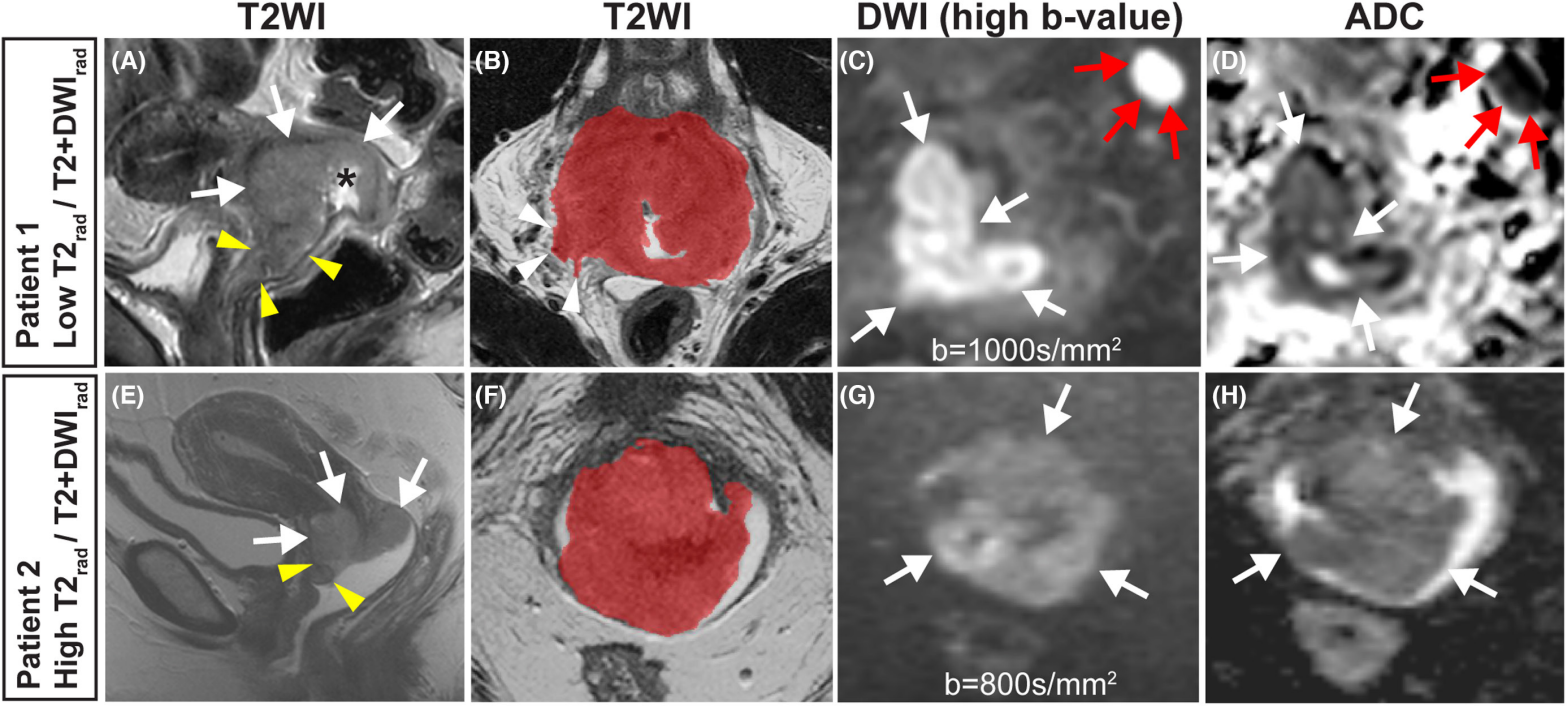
Cervical cancer depicted by sagittal T2‐weighted imaging (T2WI) (A, E), axial oblique T2WI (with manually segmented tumor mask) (B, F), axial/axial oblique diffusion‐weighted imaging (DWI) (*b* = 1000 s/mm^2^ and *b* = 800 s/mm^2^) (C, G) with corresponding apparent diffusion coefficient (ADC) maps (D, H) in two different patients diagnosed with 2018 FIGO stage IIIC1. (A–D) Patient 1: A 48‐year‐old woman having low radiomic score for the signatures T2_rad_ and T2 + DWI_rad_ and a large cervical tumor (white arrows; squamous cell carcinoma) with central ulceration (A, black asterisk). MRI‐measured maximum tumor size is 5.9 cm. The tumor extends to the upper 1/3 of the vagina (A, yellow arrowheads) and invades the parametrium (B, white arrowheads). An enlarged pelvic lymph node (short axis diameter 1.2 cm) is depicted with restricted diffusion (C, D red arrows). The primary tumor also exhibits restricted diffusion (white arrows) with hyperintensity on high *b*‐value (C), and hypointensity on the corresponding ADC map (D). The patient was subjected to primary chemoradiotherapy with no signs of recurrence 5.5 years after treatment. (E–H) Patient 2: A 47‐year‐old woman having high radiomic score for both T2_rad_ and T2 + DWI_rad_ and a large cervical tumor (white arrows; adenocarcinoma). MRI‐measured maximum tumor size is 4.8 cm, and the tumor extends to the upper part of the vagina (E, yellow arrowheads). The primary tumor exhibits restricted diffusion (white arrows) with hyperintensity on high *b*‐value (G), and hypointensity on the corresponding ADC map (H). The patient also had an enlarged pelvic lymph node (short axis diameter 1.2 cm; not shown) with restricted diffusion close to the left external iliac vessels. The patient received primary chemoradiotherapy, but experienced lymph node recurrence and died from cervical cancer 3.5 years after treatment.
